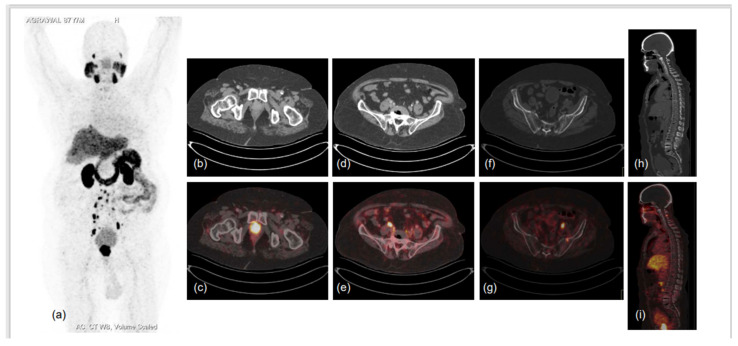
87-year-old male diagnosed with adenocarcinoma of prostate (Gleason score 7) in 2017 was heavily pre-treated: ADT with Pamorelin + Bicalutamide followed by Abiraterone and Enzalutamide. Nadir PSA was 0.016 ng/mL during the treatment and biochemical progression was observed for 6 months following. He presented to the doctor’s office with shortness of breath, limp legs, weakness and leg pain, his serum PSA was 94.7 ng/mL, and he was referred for PSMA PET CT scan. ^68^Ga-PSMA-11 PET CT scan showed prostatomegaly invading the urinary bladder wall with PSMA expressing irregular enhancing SOL involving almost entire gland. PSMA expressing multiple metastatic pelvic and retroperitoneal lymph nodes and PSMA expressing multiple sclerotic metastatic skeletal lesions showed disease progression as compared to previous PSMA PET-CT scan of 2019. (a)-MIP image, (b–i)-CT and fused PET-CT trans-axial and sagittal images.
